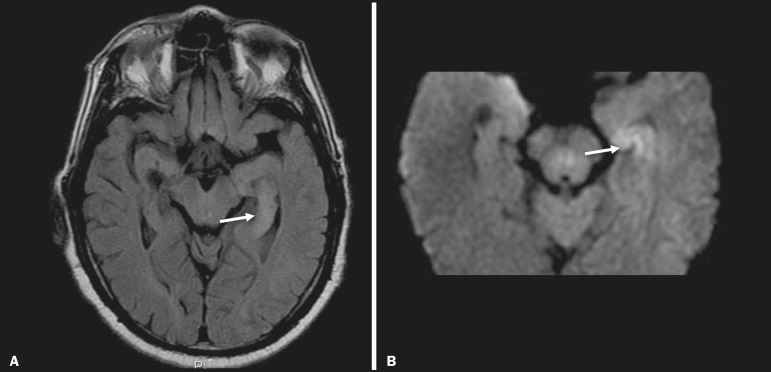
MRI of postictal edema. A: Axial FLAIR sequence showing a hyperintense signal in the left hippocampus. B: DWI showing restricted diffusion.
